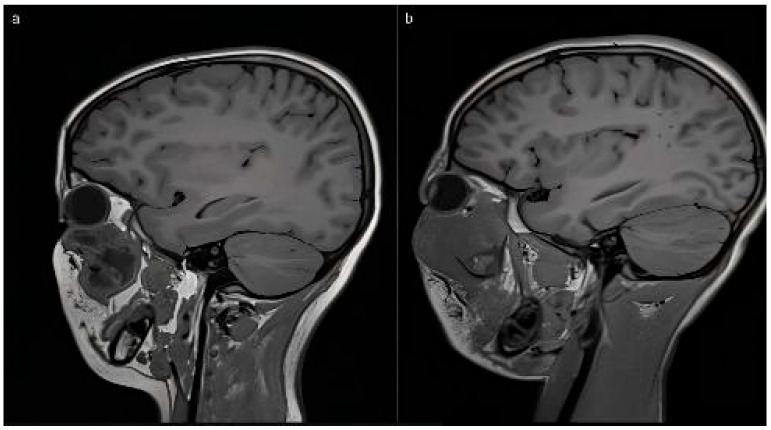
MRI images from both induced osteosarcomas affecting the maxillary sinus region (a) and the extent to the posterior orbital region and the pterygopalatine areas (b).
